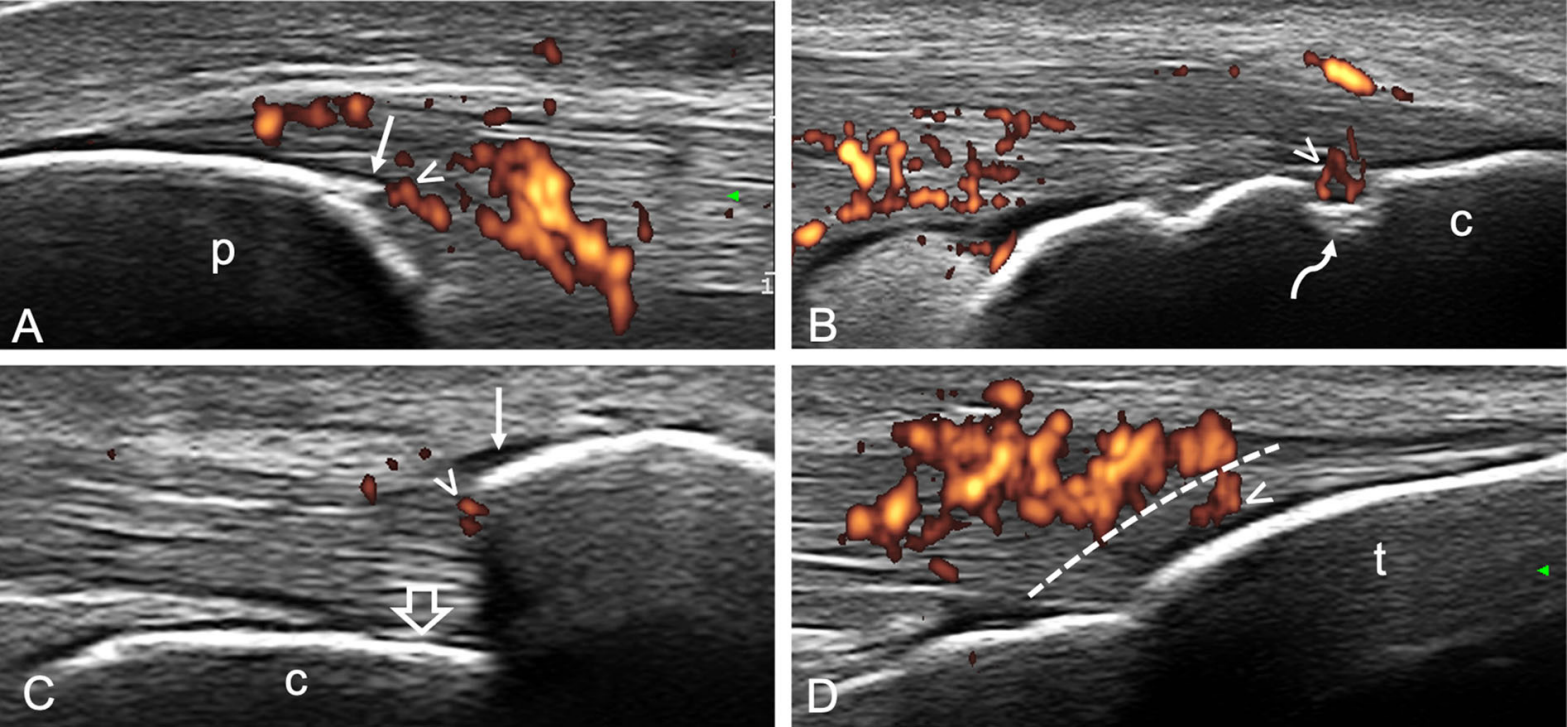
Longitudinal scans representative of enthesitis in psoriatic arthritis obtained with a 6–18-MHz probe at the proximal patellar tendon (A), Achilles tendon (B, C), and distal patellar tendon (D) insertions. Power Doppler signal (arrowhead) within 2 mm from the bony attachment is shown in A, B, C, and D. In C, power Doppler signal (score 1) is close to an exuberant enthesophyte (arrow) that disrupts the entheseal cortical line (open arrow) impairing its complete visualization. Of note, in D, only a small amount of PD signal (arrowhead) is in the region within 2 mm from the bony cortex (dashed line) even though a marked vascularization is present just proximal to it, leading to the same PD score shown in C (score 1). Note the proximity between power Doppler signal and signs of structural bone damage, such as enthesophytes (arrow) in A and C and a “hot” bone erosion (curved arrow) in B. c = calcaneus, p = patella, t = tibial tuberosity
